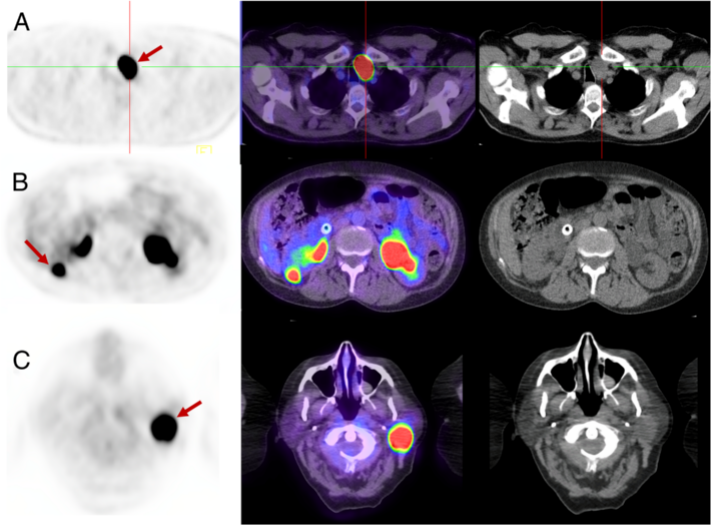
Three different patients with (a) Hurthle cell adenoma (thyroid oncocytoma), (b) renal oncocytoma and (c) Parotid Warthin’s tumour (parotid oncocytoma). Each has high SUVmax of 45, 22 and 35, respectively. In each case, the abnormality was present on imaging more than one year prior and unchanged in size. The very intense FDG uptake could be interpreted as suspicious for aggressive malignancy but the lack of temporal change was inconsistent with this. The lack of progression in a thyroid, renal or parotid lesion with very intense uptake is pathognomonic of benign oncocytomas
